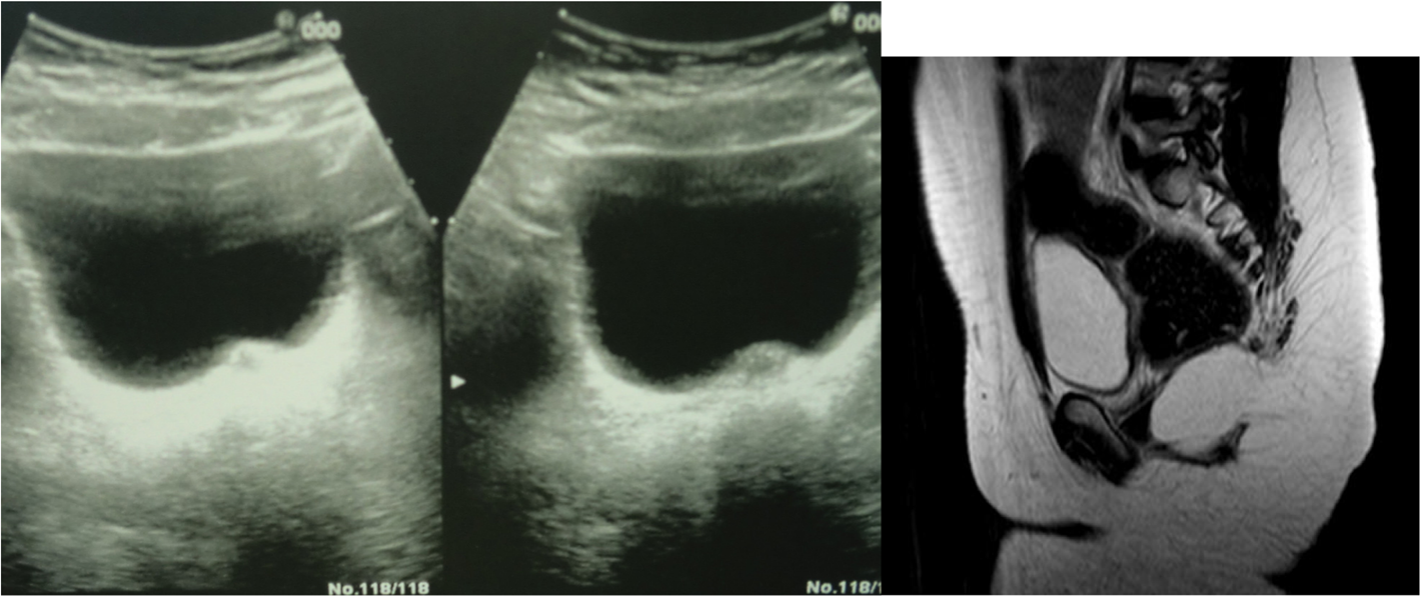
US and MRI immaging in a patient with hypoplastic uterus
